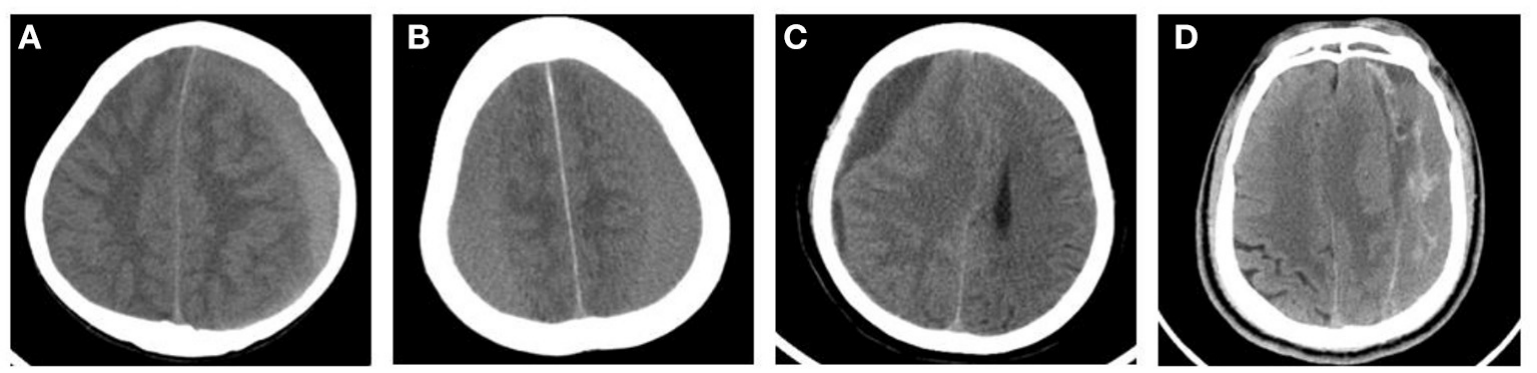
Typical CT images of the classification of CSDH according to the hematoma density. (A) High-density hematoma (HDH). (B) Iso-density hematoma (IDH). (C) Low-density hematoma (LDH). (D) Mixed-density hematoma (MDH).
